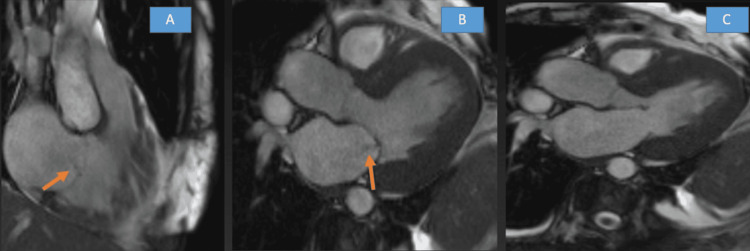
Cardiac MRI with late gadolinium enhancement images showing (A) mild to moderate tricuspid regurgitation (regurgitant fraction 33%), (B) mild to moderate mitral regurgitation (regurgitant fraction 35%), and (C) normal cardiac MRI for comparison MRI: magnetic resonance imaging
